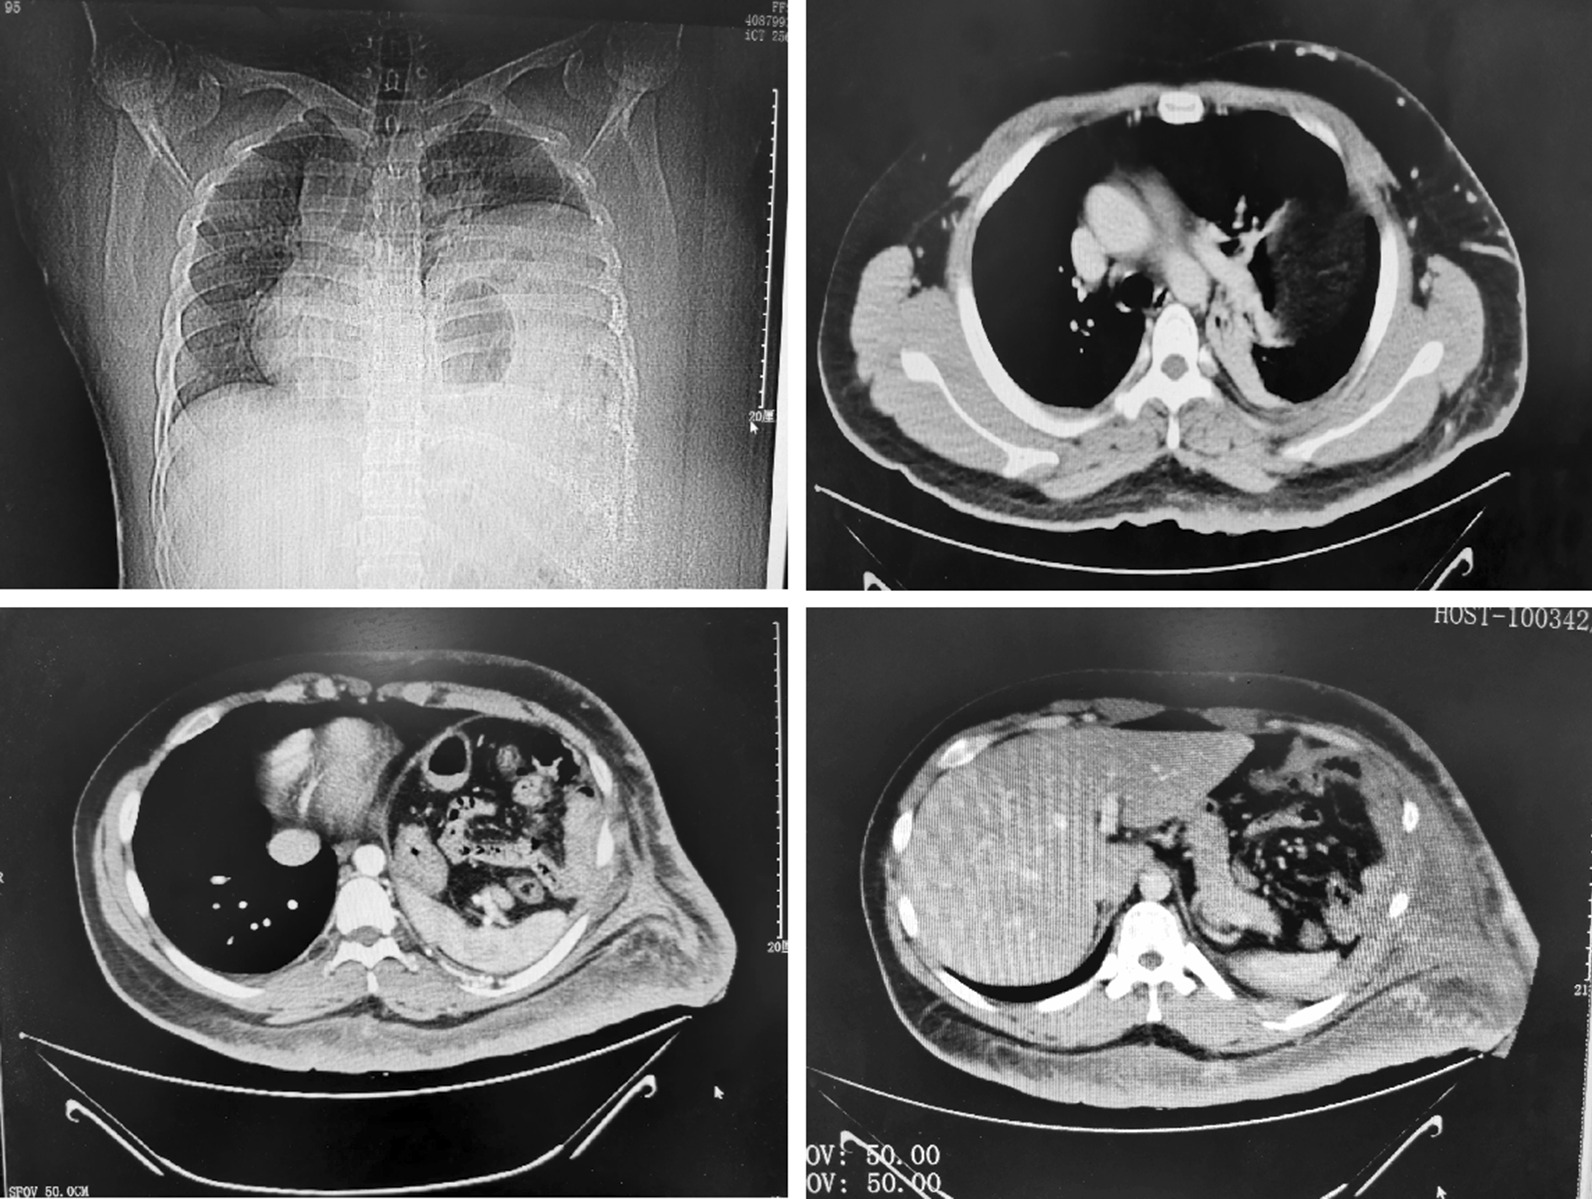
Chest X-ray and chest CT scan at admission
